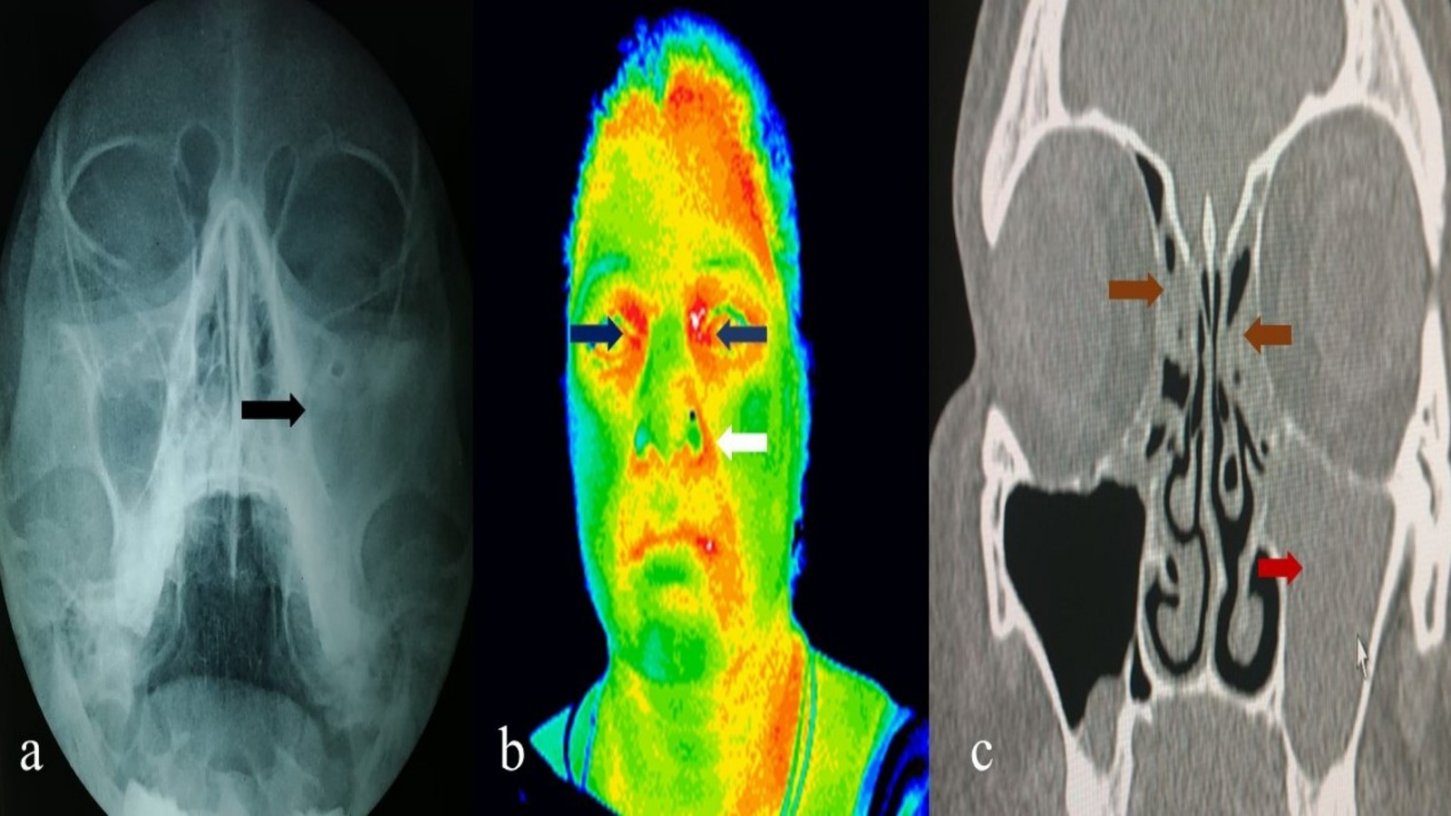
X-ray nose and paranasal sinuses (a) showing haziness in the left maxillary sinus (black arrow) and ethmoidal regions. Thermography of head and neck (b) showing regions of hyperthermia in the left nasolabial fold (white arrow) and bilateral ethmoidal regions (blue arrow) suggestive of left maxillary and bilateral ethmoidal sinusitis. Computed tomography of nose and paranasal sinuses (c) showing hyperdensity in left maxillary (red arrow) and bilateral ethmoidal sinuses (brown arrow)
